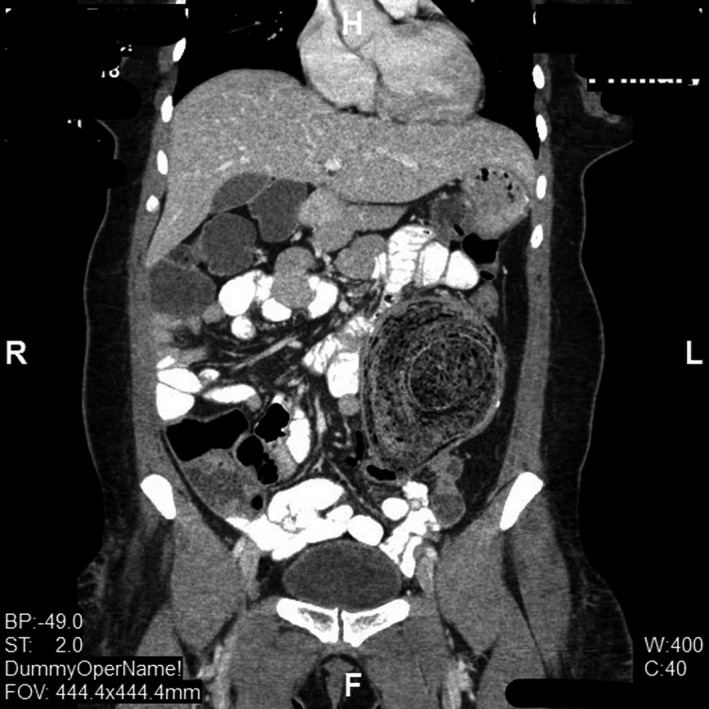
demonstrates coronal section of the CT scan of abdomen with the large fecaloma. Arrow indicates the leading loop of colo‐colonic intussusception
